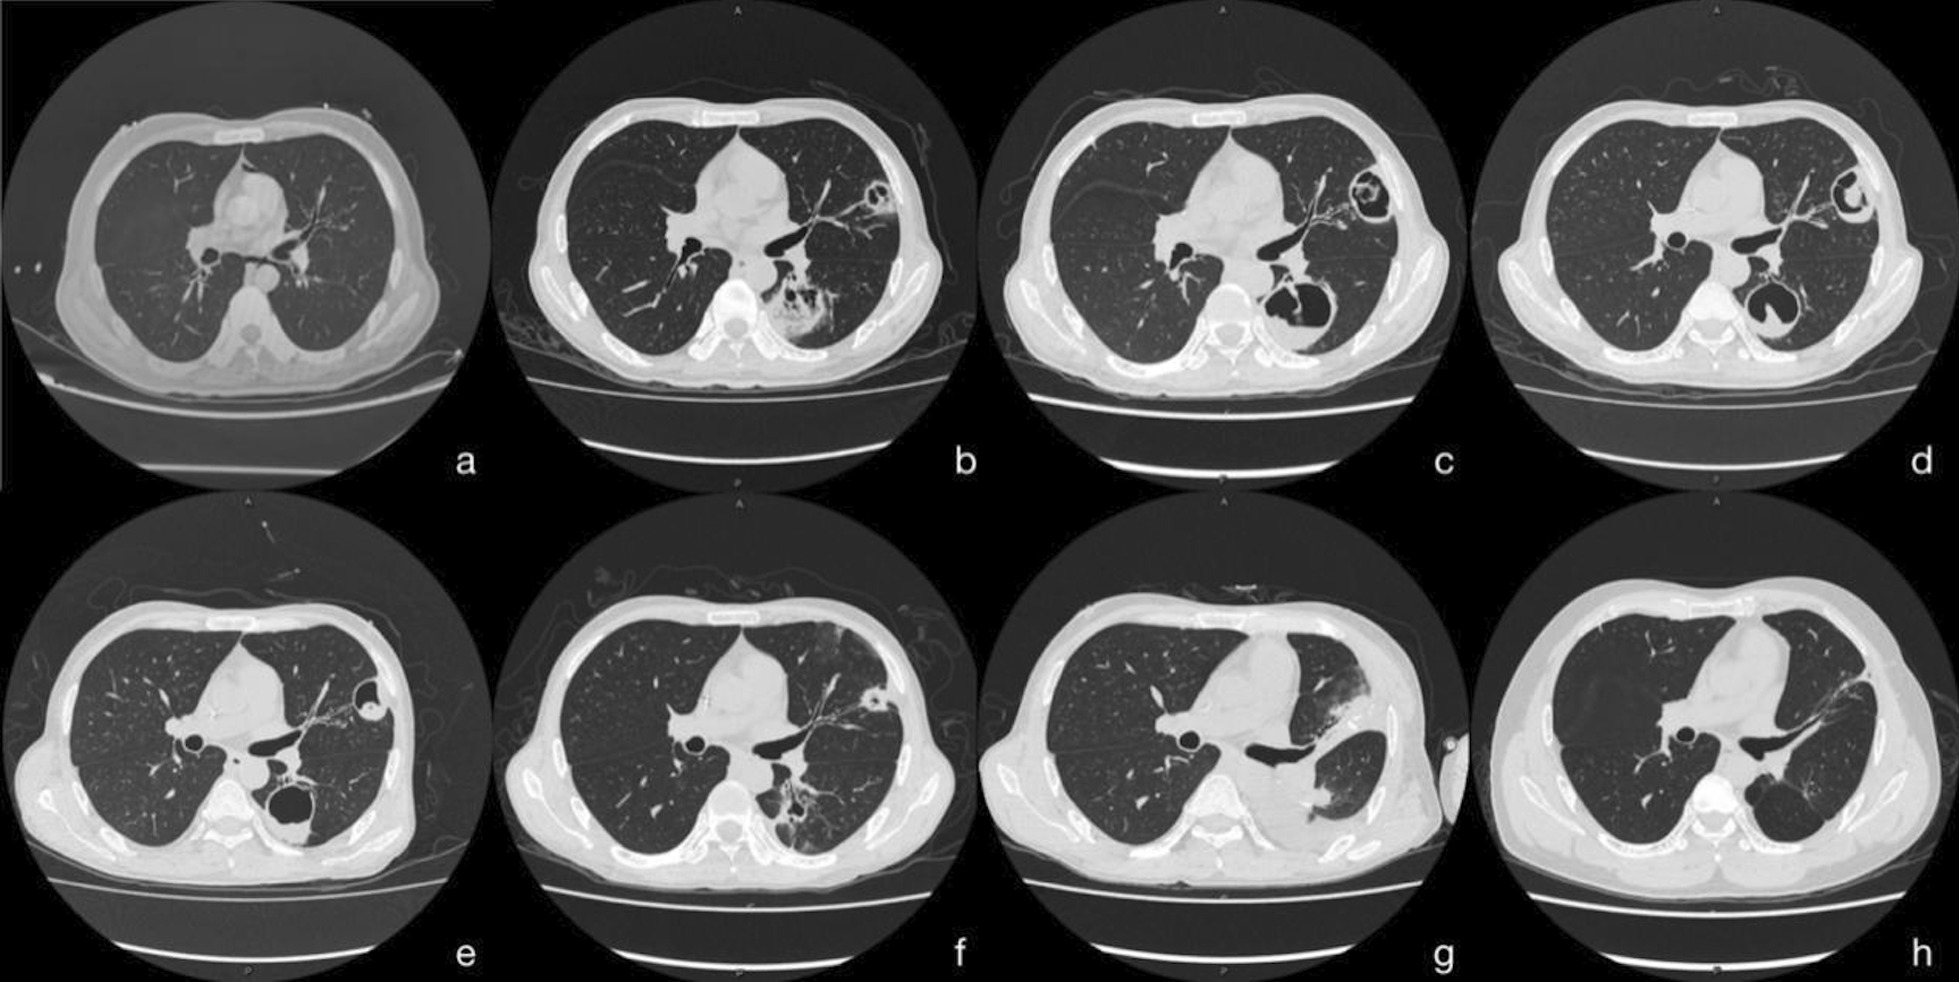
a Chest CT showed no abnormalities before admission to our hospital. b PET-CT showed cavitary lung lesions on day 13 after admission. c Chest CT showed that the cavitary lung lesions were slightly larger than before on day 23 after admission. d chest CT revealed increased consolidation in the cavitary lung lesions on day 37 after admission. e chest CT revealed that the cavitary lung lesions were smaller than before on day 47 after admission. f chest CT showed that the lung cavities still existed, and the exudates around the lesion got slightly reduced on day 127 after admission. g chest CT showed that the upper lingual and dorsal left lower lobe lesions of the left upper lobe were removed 10 days after operation (on day 151 after admission). h chest CT showed no new infections after 3 years follow-up
